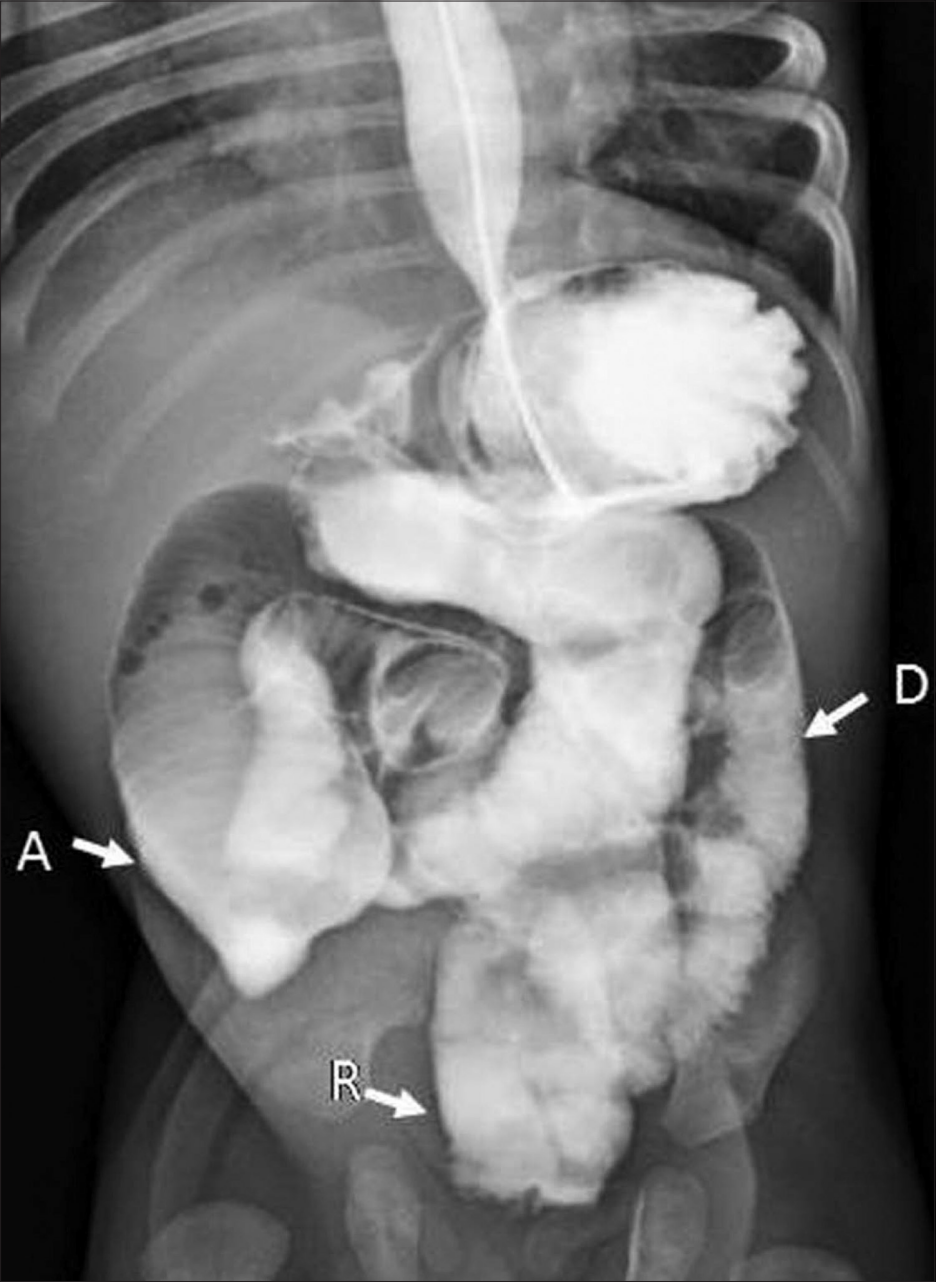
Frontal radiograph from a barium meal follow-through study shows contrast-opacified short length of dilated small bowel; A, ascending colon; D, descending colon, R, rectum. Rapid transit was noted, with contrast seen in the large bowel within 20 minutes of administration (normal mean transit time is 60 minutes)
